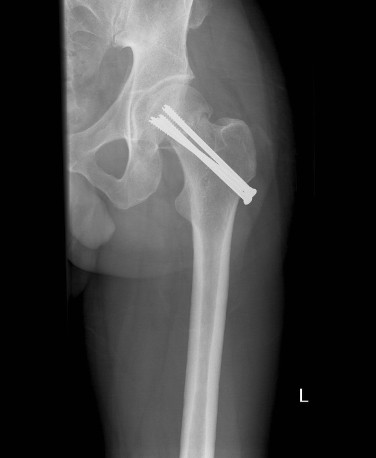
Radiograph of his left hip six months after surgery.
